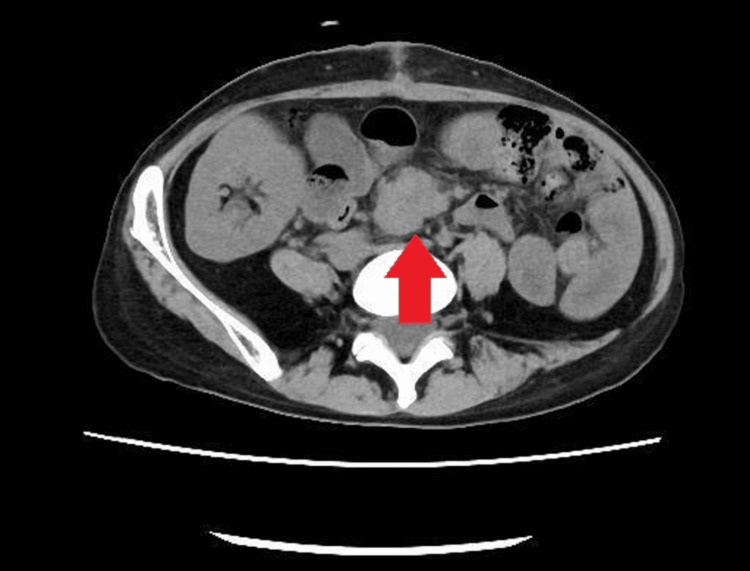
Computed Tomography Showing Disease Progression After Surgical Resection Postoperative CT reveals a newly developed mesenteric mass (arrow) consistent with recurrent diffuse large B-cell lymphoma prior to initiation of systemic chemotherapy.
